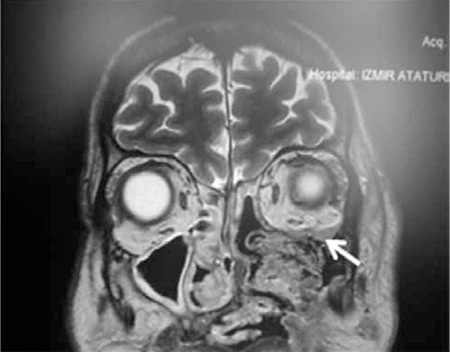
Case 4, magnetic resonance imaging images of patient number 4 who underwent left maxillectomy and hard palate resection. The area of suspected involvement in the inferior wall orbital wall is shown with a white arrow
